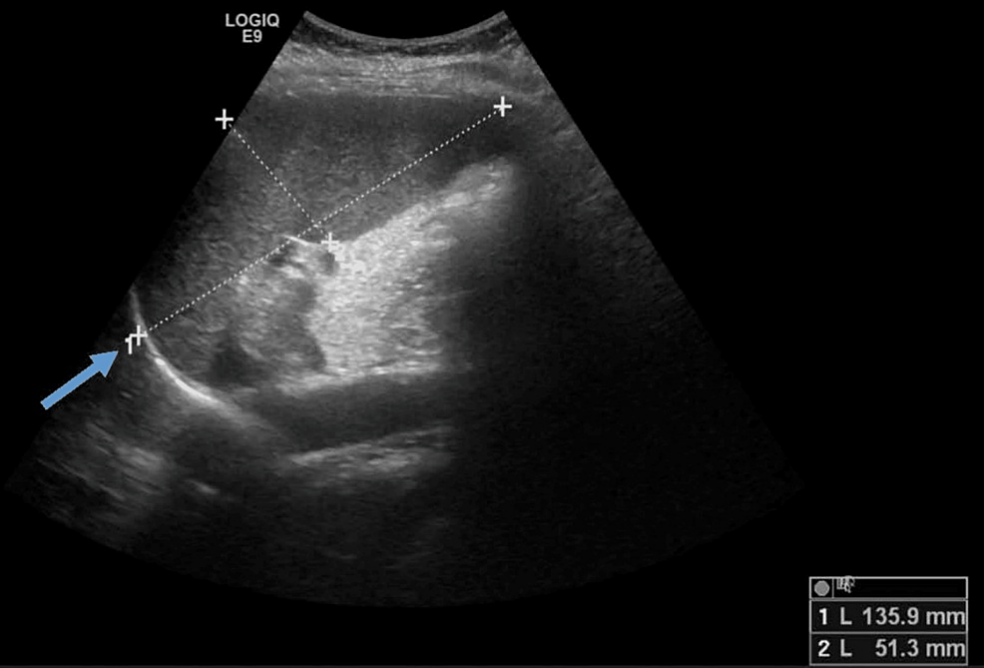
Splenomegaly on abdominal ultrasound Splenomegaly was confirmed in the abdominal ultrasound. The blue arrow identifies the splenic length axis of 135.9 mm.
